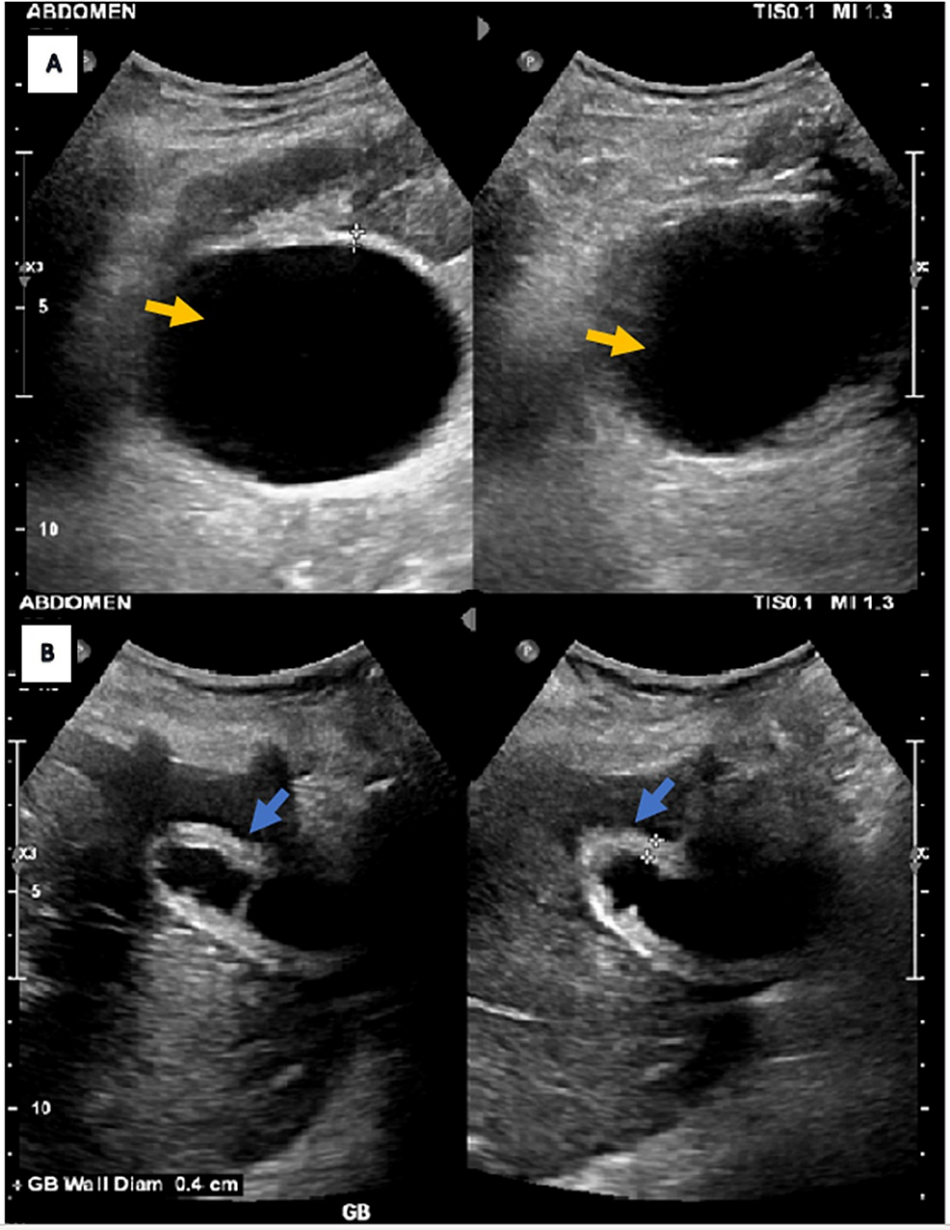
Ultrasound abdomen (A) Yellow arrows show a grossly distended gallbladder (B) Blue arrows show thickened wall measuring 4 mm
